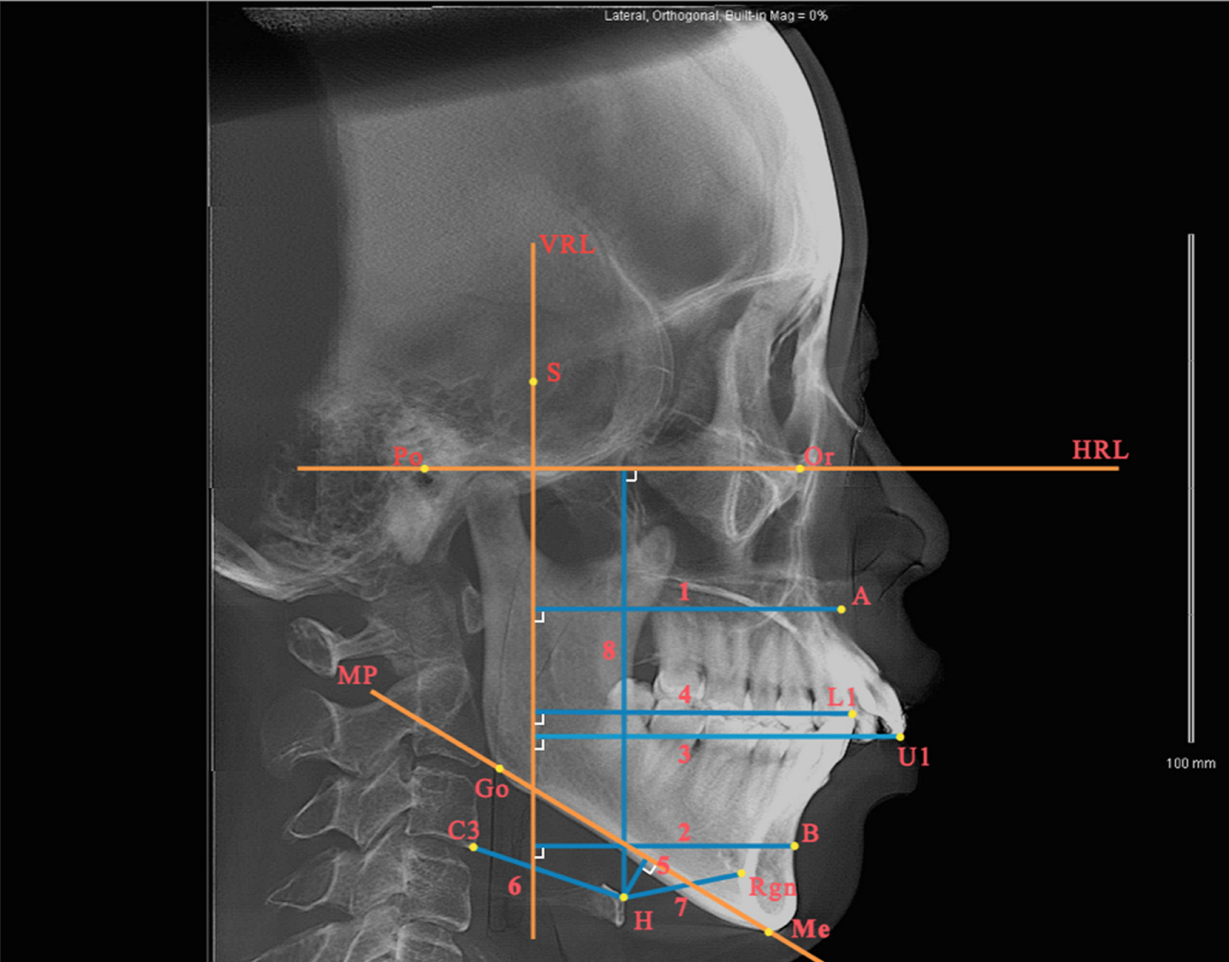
Measurements of the craniofacial structures and hyoid position in the lateral cephalograms generated by CBCT. (1) A-VRL; (2) B-VRL; (3) U1-VRL; (4) L1-VRL; (5) H-MP; (6) H-C3; (7) H-Rgn; and (8) H-HRL.
